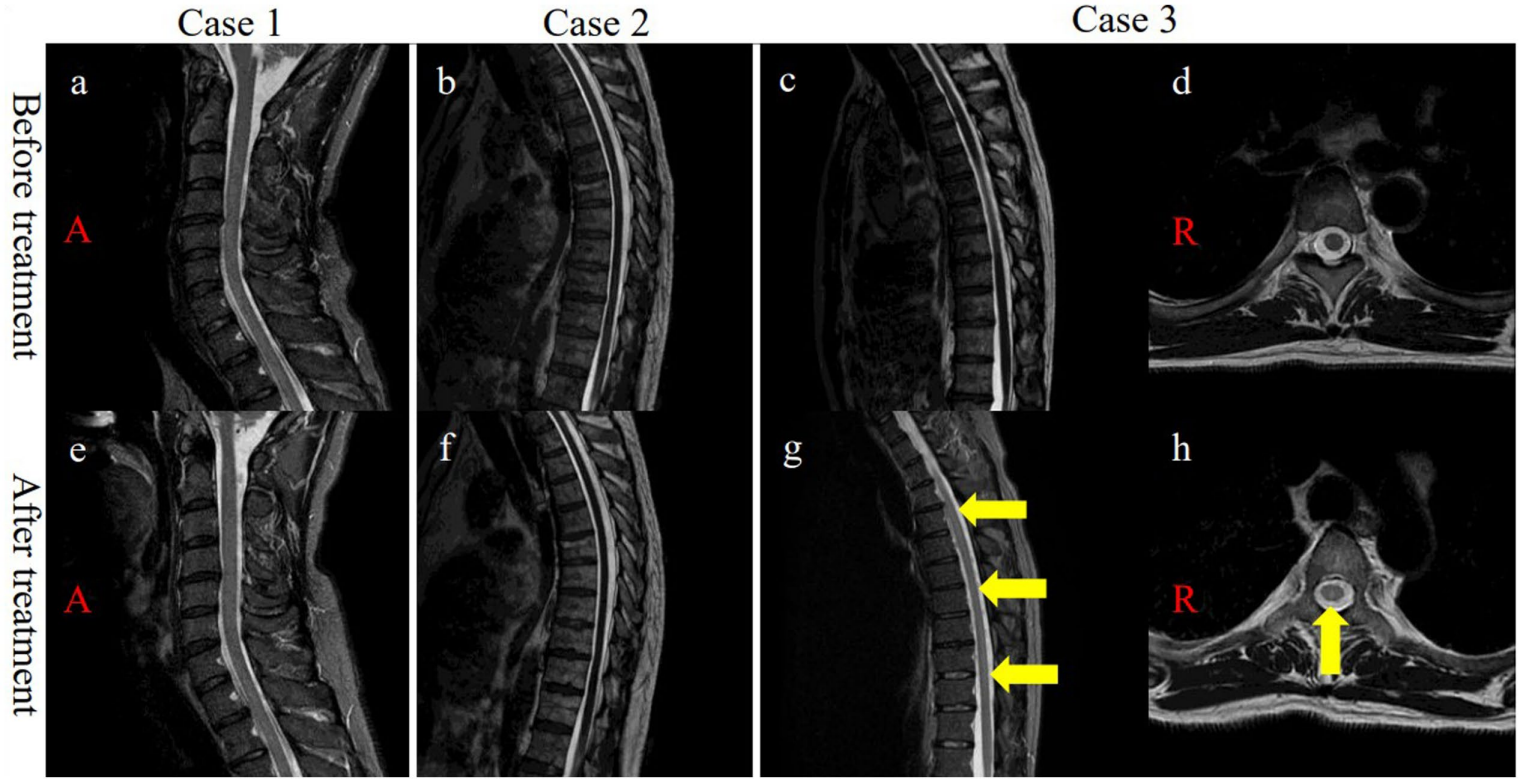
(A) Cervical and (B,C) thoracic initial MRI displayed no signal changes on sagittal T2WI. (D) Axial T2WI of T5 showing no signal changes. (E) No signal abnormalities were observed in the cervical cord after treatment. (F) No signal changes were observed in the thoracic cord after treatment. (G) Post-treatment MRI demonstrated signal changes in the dorsal columns (T1-T10) on sagittal STIR (yellow arrows, g). (H) Post-treatment MRI confirmed signal changes in the thoracic dorsal columns (T5) on axial T2WI (yellow arrow, h). MRI, magnetic resonance imaging; T, thoracic vertebrae, T2WI, T2-weighetd imaging; STIR, short T1 inversion recovery.
